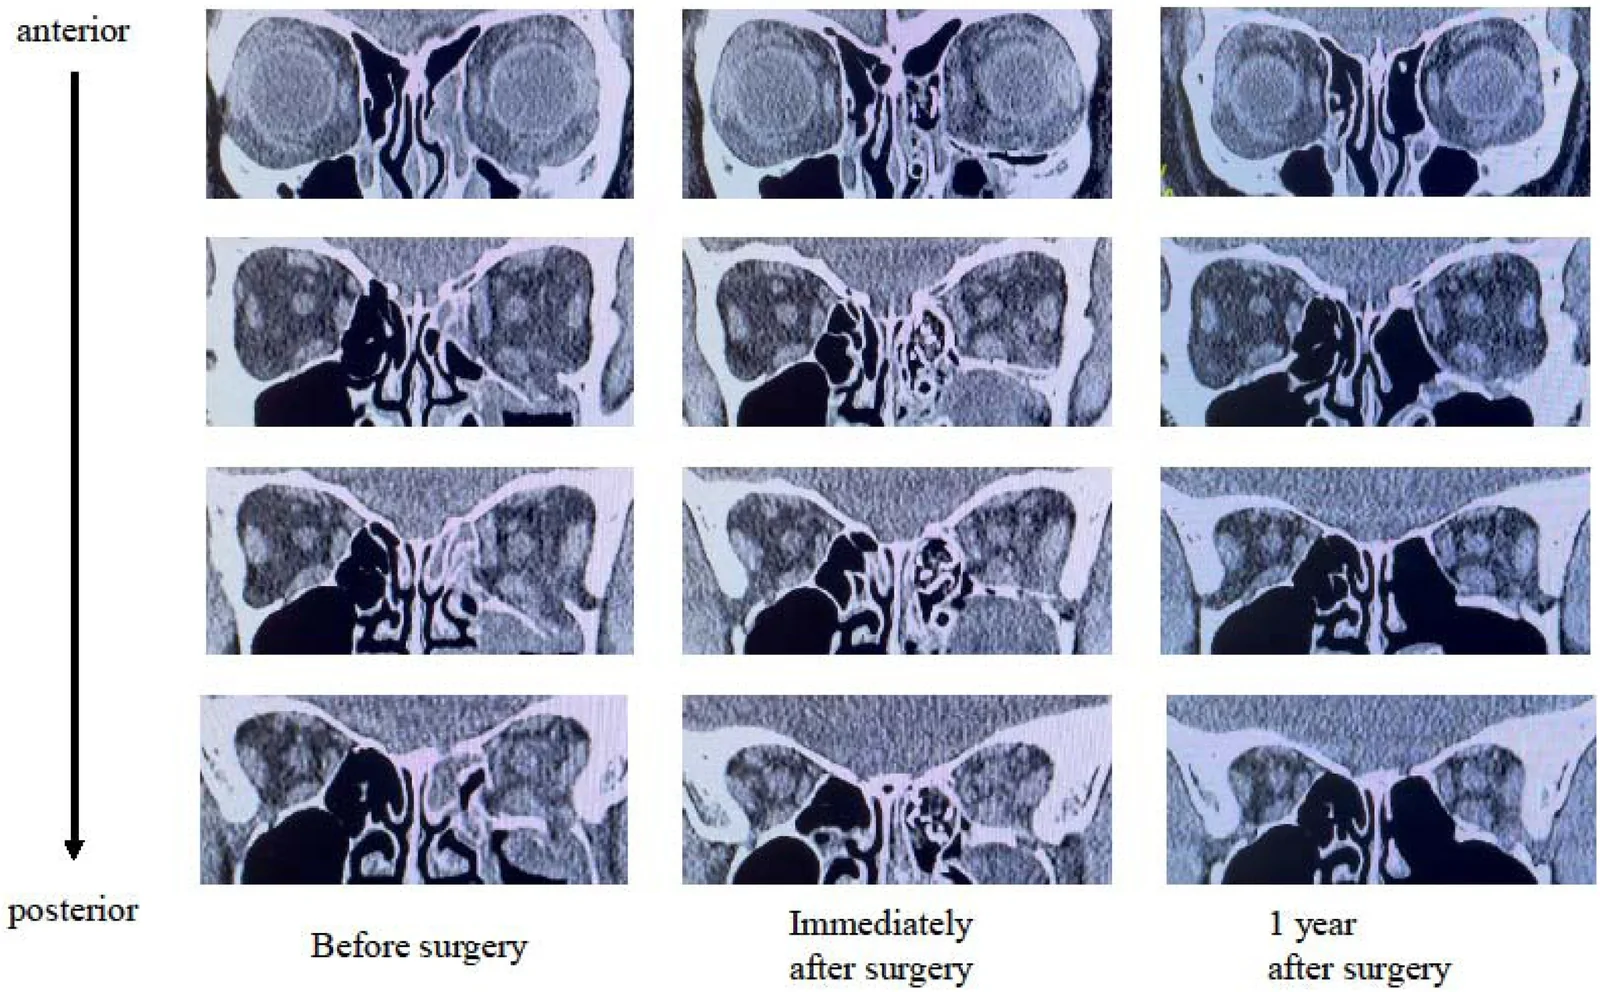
Computed Tomography (CT) images of the DSP before, immediately after surgery, and 1-year after surgery. Coronal CT images are arranged in three columns representing the preoperative (left), immediate postoperative (middle), and 1-year postoperative (right) time points. At each level, both orbits are shown on the same slice to allow comparison of vertical orbital position. Images are ordered from anterior (top row) to posterior (bottom row). Following DSP, the bony orbital floor was reconstructed, and the medial wall contour was maintained by a fibrous capsule formed along the orbital medial wall.
